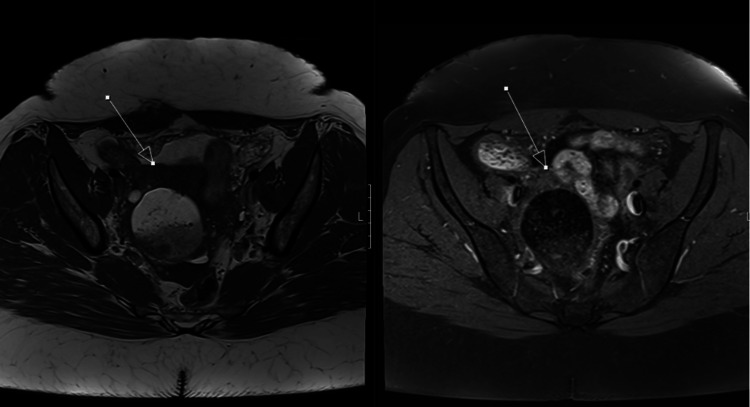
MRI Pelvis Showing Residual Dermoid Cyst and Capsular Discontinuity Axial T1-weighted (left) and T1 fat-saturated (right) images of the pelvis demonstrating a right-sided dermoid cyst with an internal fat-fluid level. Additionally, a fat–fluid level can be visualised within the peritoneal cavity between bowel loops (arrow), consistent with rupture.
